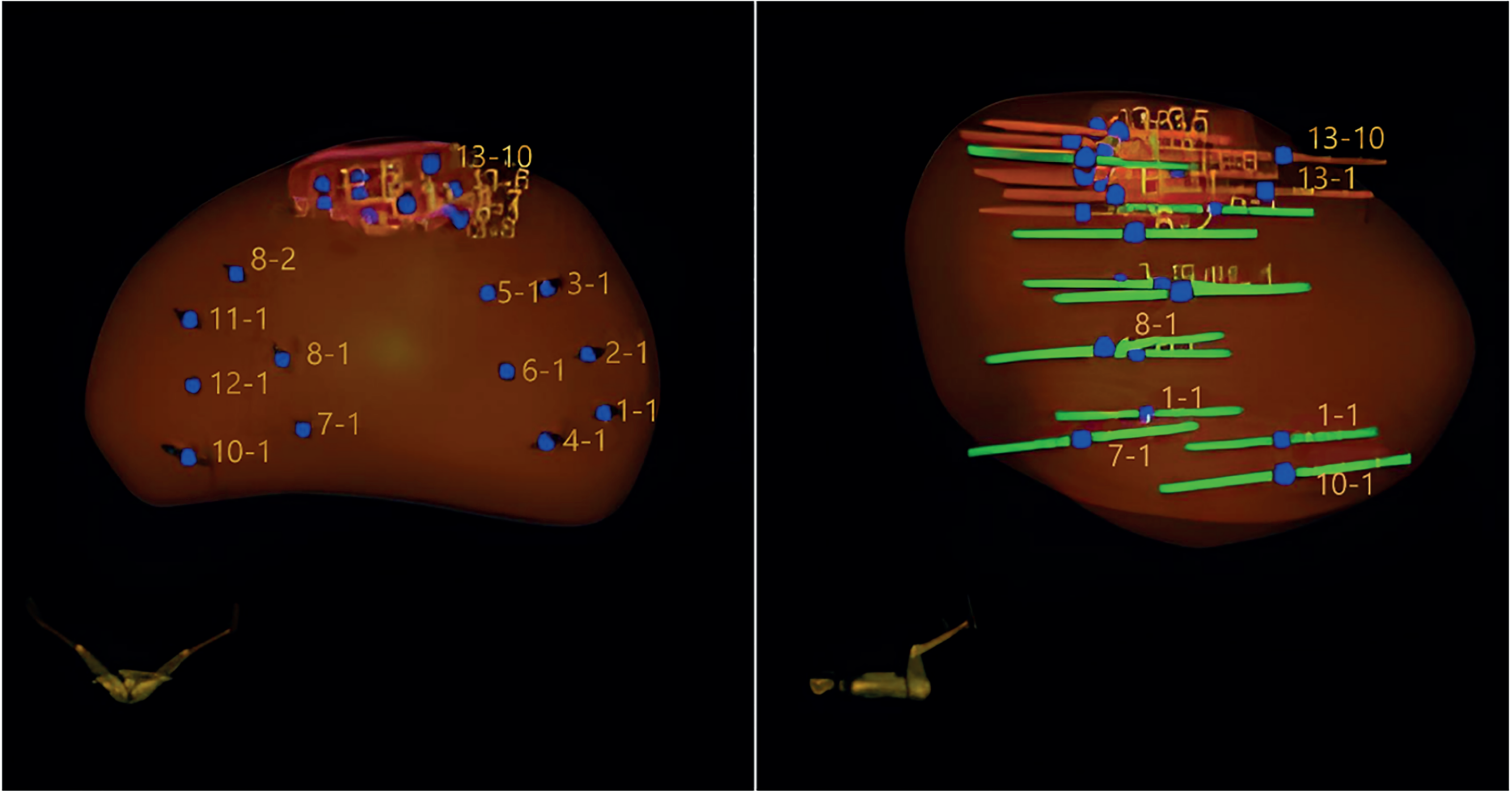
Biopsy method. Cores were obtained from a systematic template and MRI lesion.
